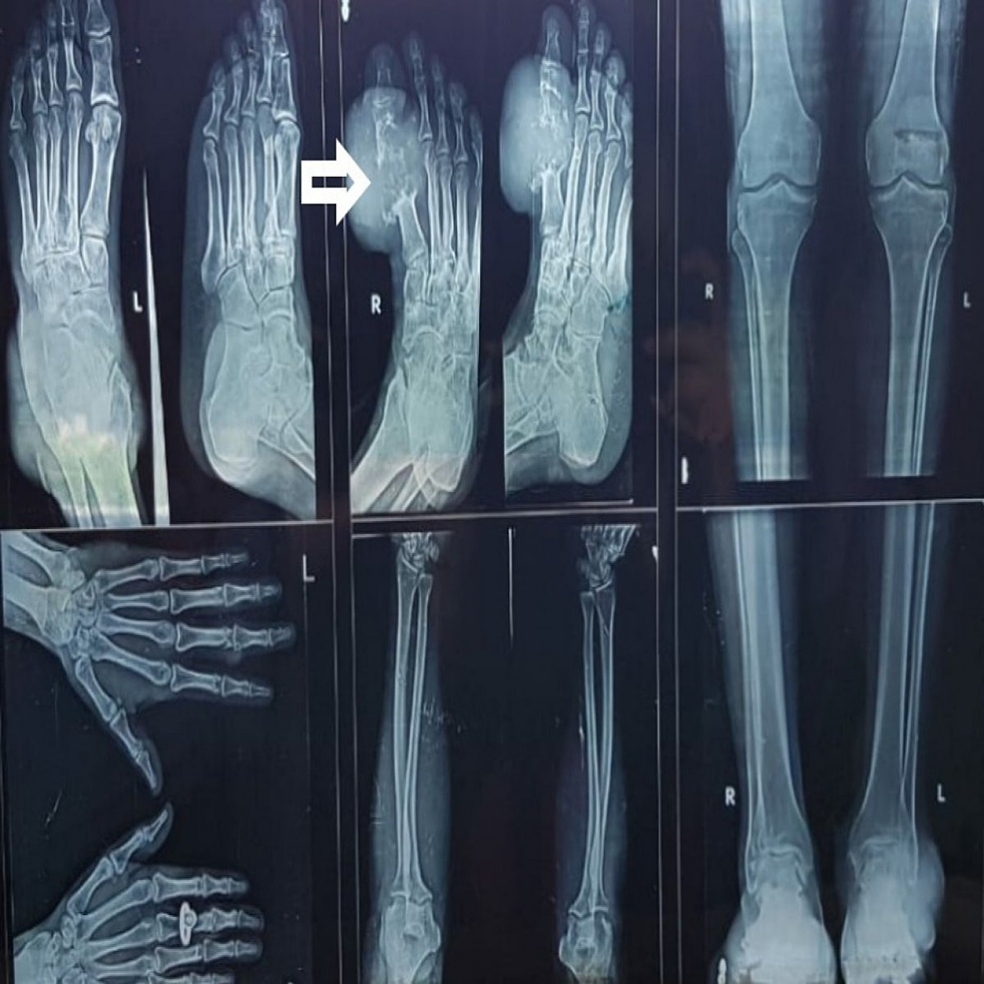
Plain radiographs of left foot, right foot, bilateral elbows left hand bilateral below knee bilateral ankle joints- revealing increased soft tissue thickness with minimal distal acoustic enhancement
